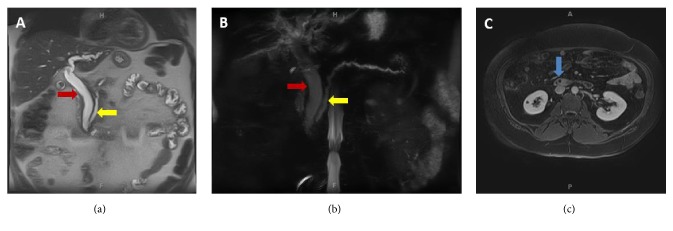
MRI abdomen with and without contrast: (a) T2 image and (b) MRCP image reveal moderate to severe intrahepatic biliary ductal dilation. The common duct measures 1.5 cm in greatest diameter “red arrow”. The pancreatic duct is also dilated measuring up to 0.6 cm at the head “yellow arrow”. No intraluminal filling defect. (c) T1 image with contrast reveals mild distal common bile duct wall enhancement “blue arrow”.
